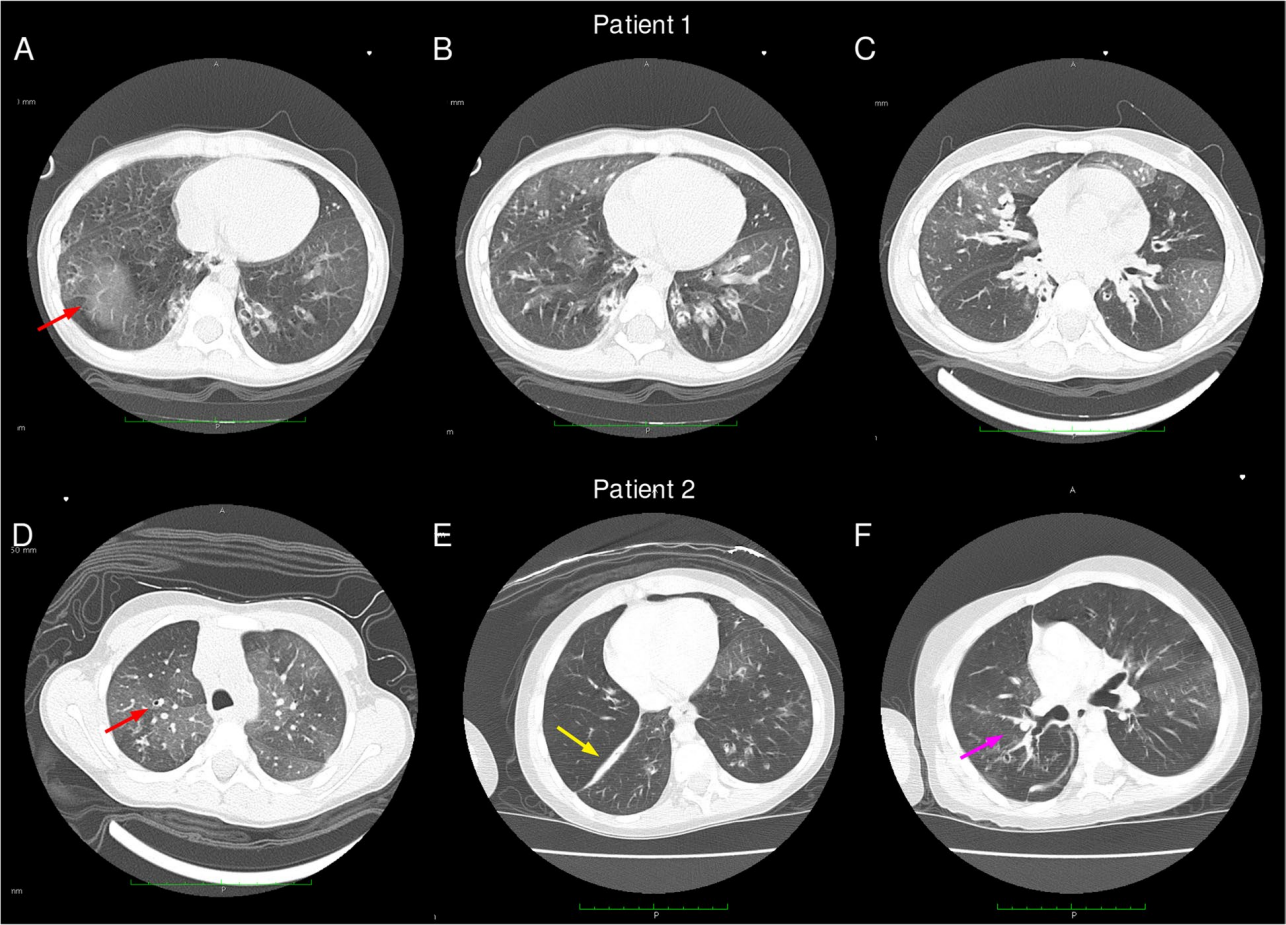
Thorax CT imaging of the two study subjects. Upper and lower rows indicate patient 1 (a-c) and patient 2 (d-f) images, respectively. a-c) was obtained in 2016 at 3 y.o of P1. Chest CT scan in d) was obtained in 2015 at 3y7mo, whereas e and f were taken in late 2017 at 6 y.o. Red arrows indicate mosaic attenuation patterns and Bronchiectasis. Yellow arrow shows atelectasis. In pink, we showed a large bronchiectasis and bronchial wall thickening
